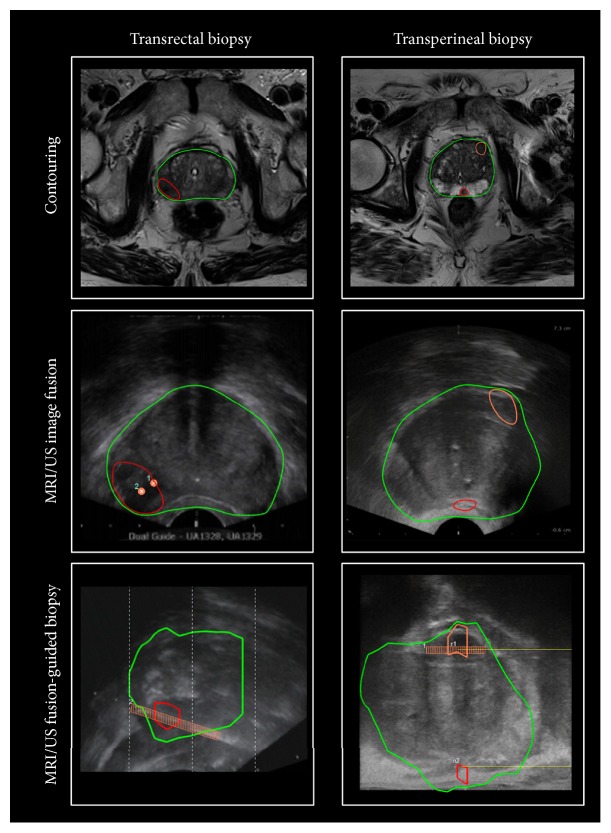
Workflow of MRI/TRUS fusion-guided biopsy with the BioJet software*. Depicted are two examples of MRI/TRUS fusion-guided biopsy, one with the transrectal (left column) approach and one with the transperineal (right column) approach. First row: contouring of the prostate margins (green) and target lesions (red and orange). Second row: fusion of MRI contours and ultrasound images in the transverse orientation. Third row: obtaining samples from the target lesion. Fusion of MRI contours and ultrasound images are shown in the sagittal orientation. Biopsy cores are marked in orange.
